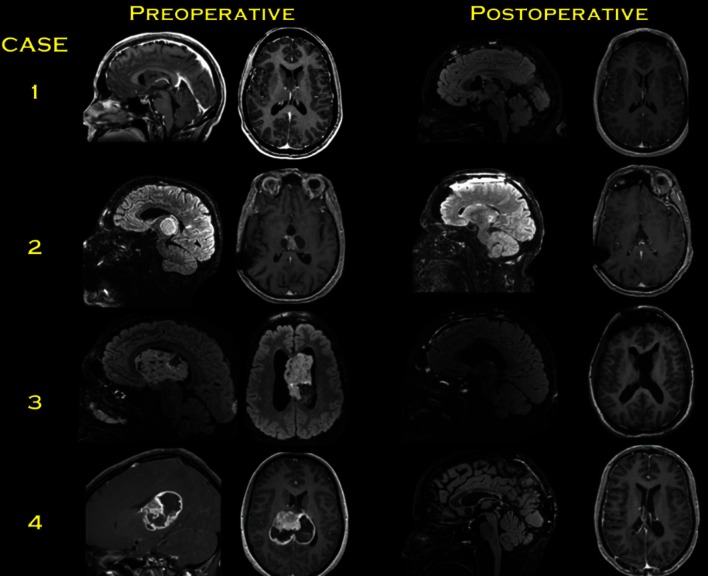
Preoperative and postoperative MR images of the cases which are presented in the complementary videos of the article. *Case 1* Sagittal and axial MRI with contrast show non-enhancing right LV tumor. Post-operative sagittal flair imaging shows the minimal callosotomy and axial post-contrast T1 imaging confirms gross total resection through interhemispheric transcallosal approach. [Please see the video 1]. *Case 2* Sagittal flair MRI and axial post-contrast T1 MRI shows a heterogeneously enhancing cystic tumor in the posterior TV. Post-operative sagittal cube MRI and post-contrast axial T1 MRI confirms gross total removal through this approach. [Please see the video 2]. *Case 3* Sagittal and axial flair MRI show a tumor occupying the frontal horn, body and atrium of the LV. Post-operative sagittal flair MRI show the extent of the callosotomy and axial T1 MRI confirms the gross total removal. [Please see the video 3]. *Case 4* The extent of heterogeneously enhancing tumor originating from the thalamus and the peripheral edema due to mass effect are shown in the contrasted sagittal and axial MRI. Postoperative sagittal flair MRI and post-contrast axial MRI confirm the gross total resection via posterior interhemispheric approach. [Please see the video 4]
